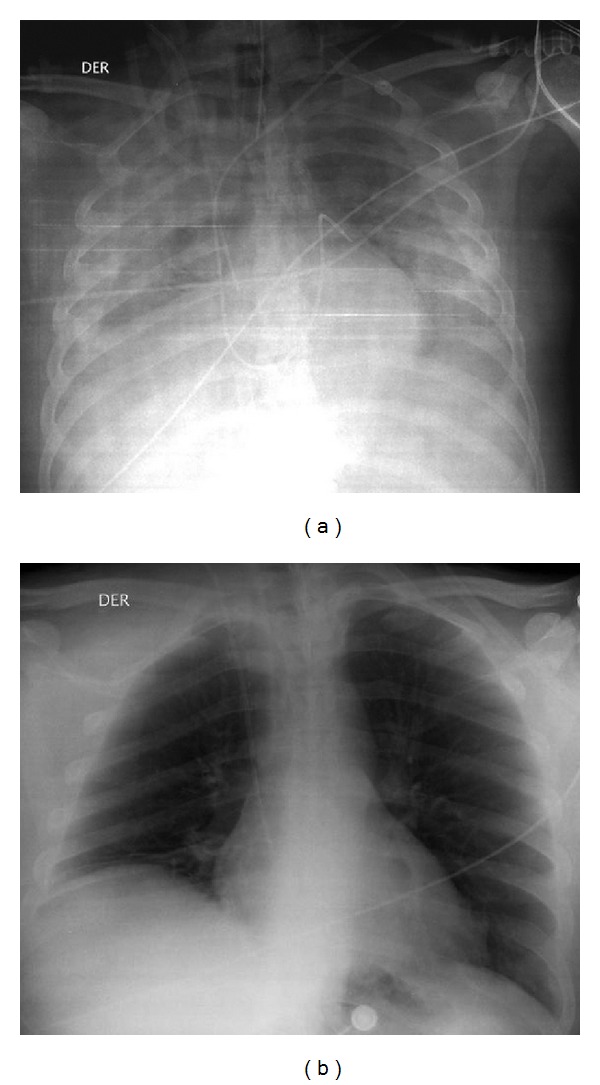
Chest imaging evolution. Chest X-ray on day 2 shows opaque lung fields (a) with resolution of infiltrates an normalization of aeration a week later (b).
